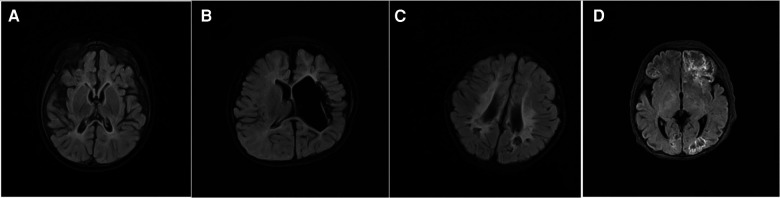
Some typical magnetic resonance imaging (MRI) findings in the patients with infantile epileptics spasms syndrome (IESS), T2 weighted imaging (T2 WI flair), (A) MRI (8-month-old) showed bilateral occipital encephalomalacia, brain atrophy, hyperintensity in the external capsule. (B) MRI (17-month-old) showed bilateral periventricular leukomalacia. (C) MRI (7-month-old) showed left hemisphere parenchyma, and left ventricular dilatation. (D) MRI (10-day-old) exhibited fronto-temporo-parietal-occipital encephalomalacia.
